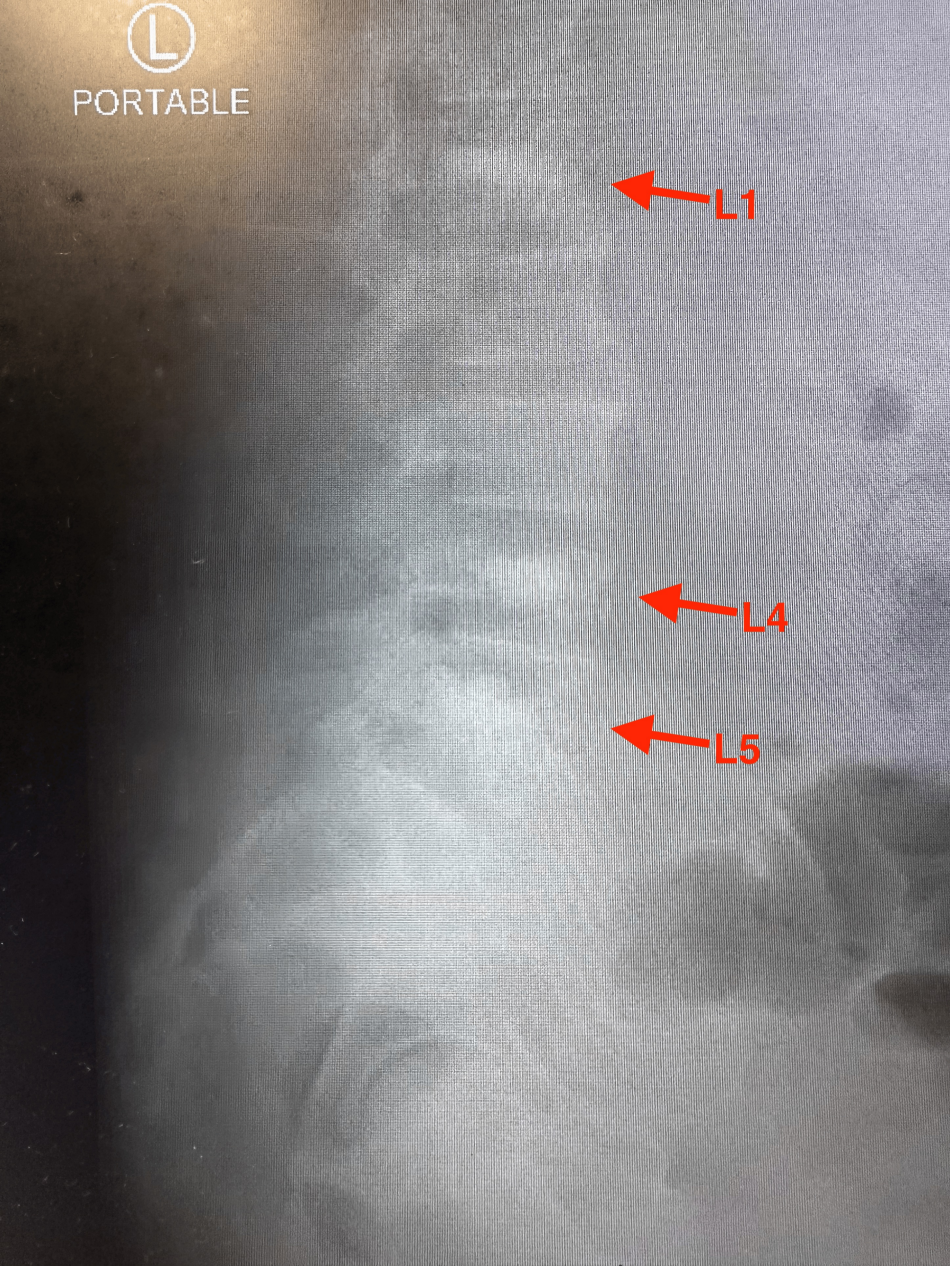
Portable lateral lumbar spine X-ray demonstrating compression deformities at L1, L4, and L5 (red arrows). Images captured using a smartphone camera due to limitations in accessing downloadable files. All identifiable information was removed.
